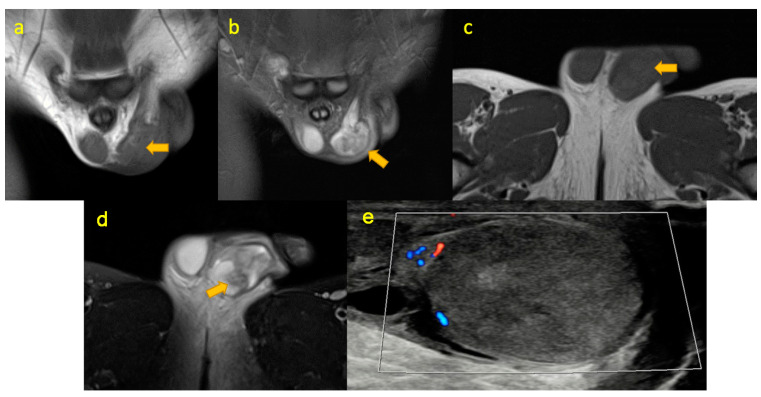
(a–d) Acute torsion (arrow) of the left-sided testicle in a 13-year-old boy with developmental delay who presented with a painful left-sided limp. Coronal T1W, coronal STIR, axial T1W, and axial T2W FS MRI images show asymmetric enlargement and heterogeneity of the left testicle. Note subcutaneous soft tissue oedema in the left hemiscrotum. (e)—colour Doppler ultrasound image in the longitudinal plane shows heterogeneous echogenicity of the left testicle and absence of normal blood flow consistent with acute torsion.
